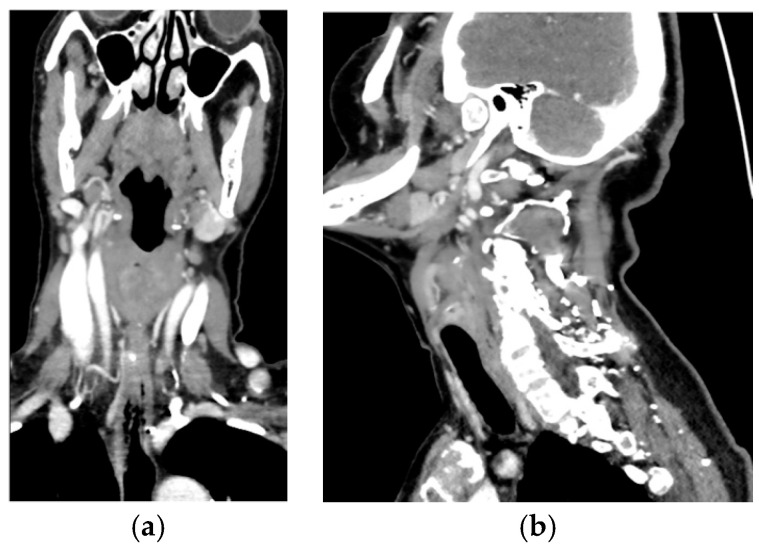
(a,b) The same case examined with 4D-CT shows the parathyroid pedicle originating from the upper cervical sections, confirming the embryological origin of the superior parathyroid (PIV). Moreover, 4D-CT has also proven to be very effective in the localization of the parathyroid of median retroesophageal location because it allows identification of the side of the vascular pedicle, facilitating the minimally invasive approach even in such circumstances. This is because this imaging technique can easily identify a parathyroid, even in an abnormal position, but it is not certain that this is really the pathological one because this is not functional imaging. Therefore, it is always necessary to combine it with MIBI scintigraphy or FCH-PET [42].
